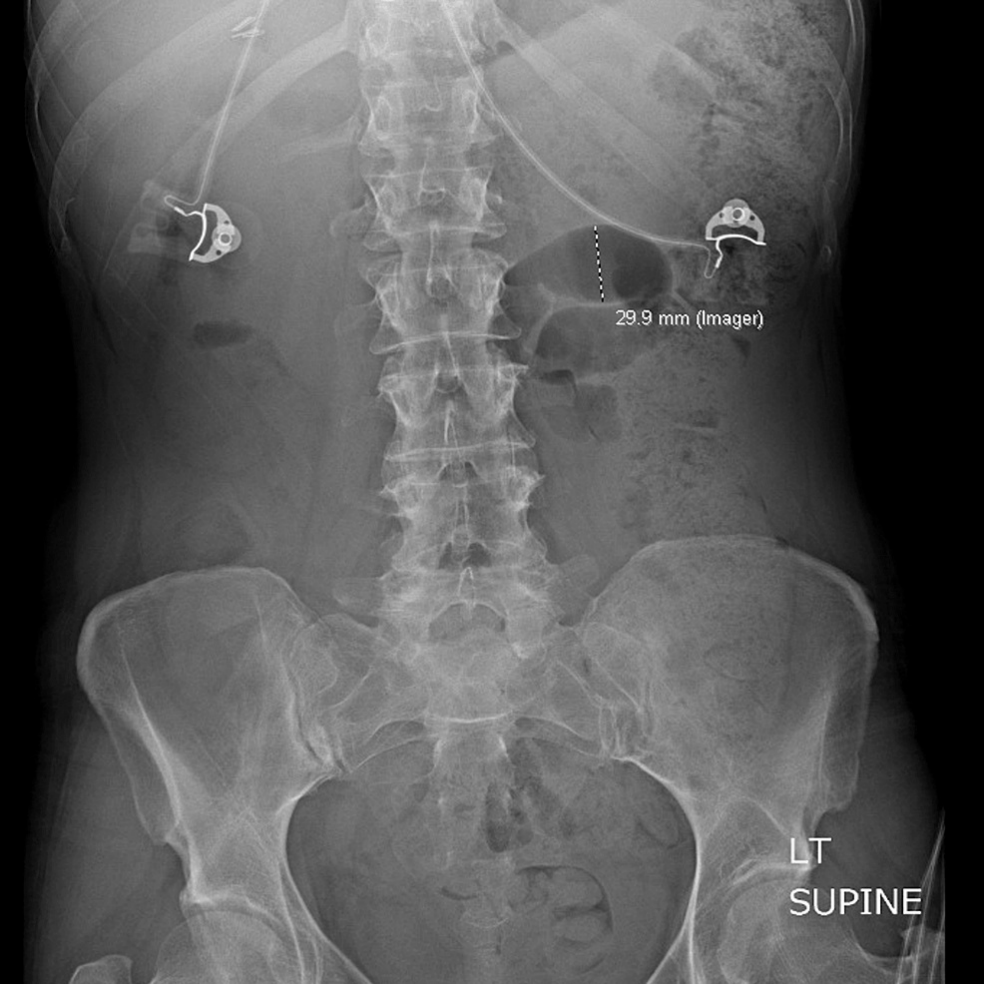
Abdominal X-ray revealing a focal dilated gas-filled loop of the small bowel in the left hemiabdomen measuring up to 3.0 cm, possibly reflecting focal ileus.
